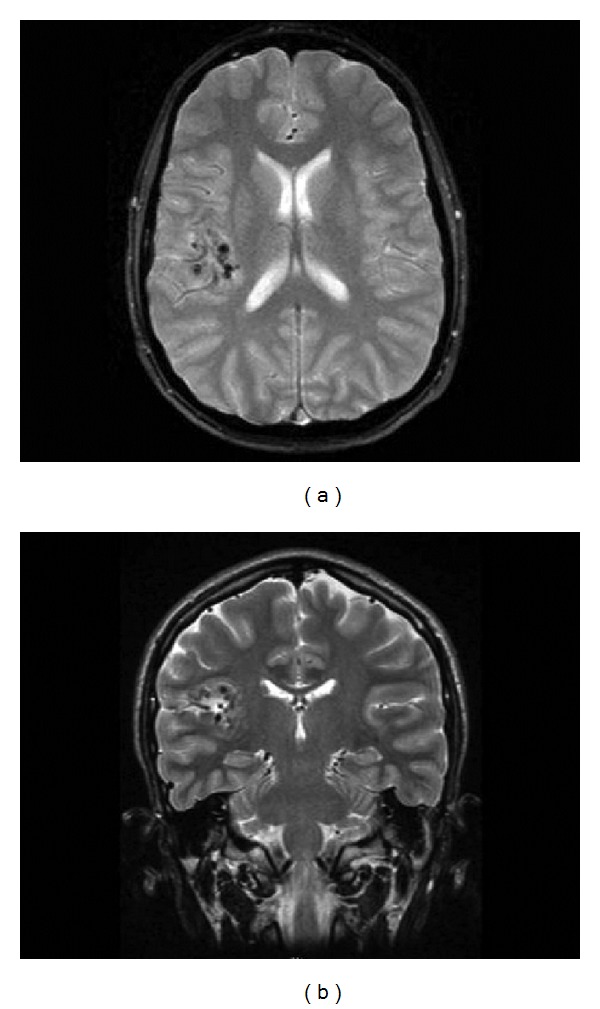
T2-weighted axial (a) and coronal (b) MRI performed 1 year prior to surgery show right posterior frontal flow void with adjacent cortical foci of susceptibility artifact suggesting vascular malformation.
